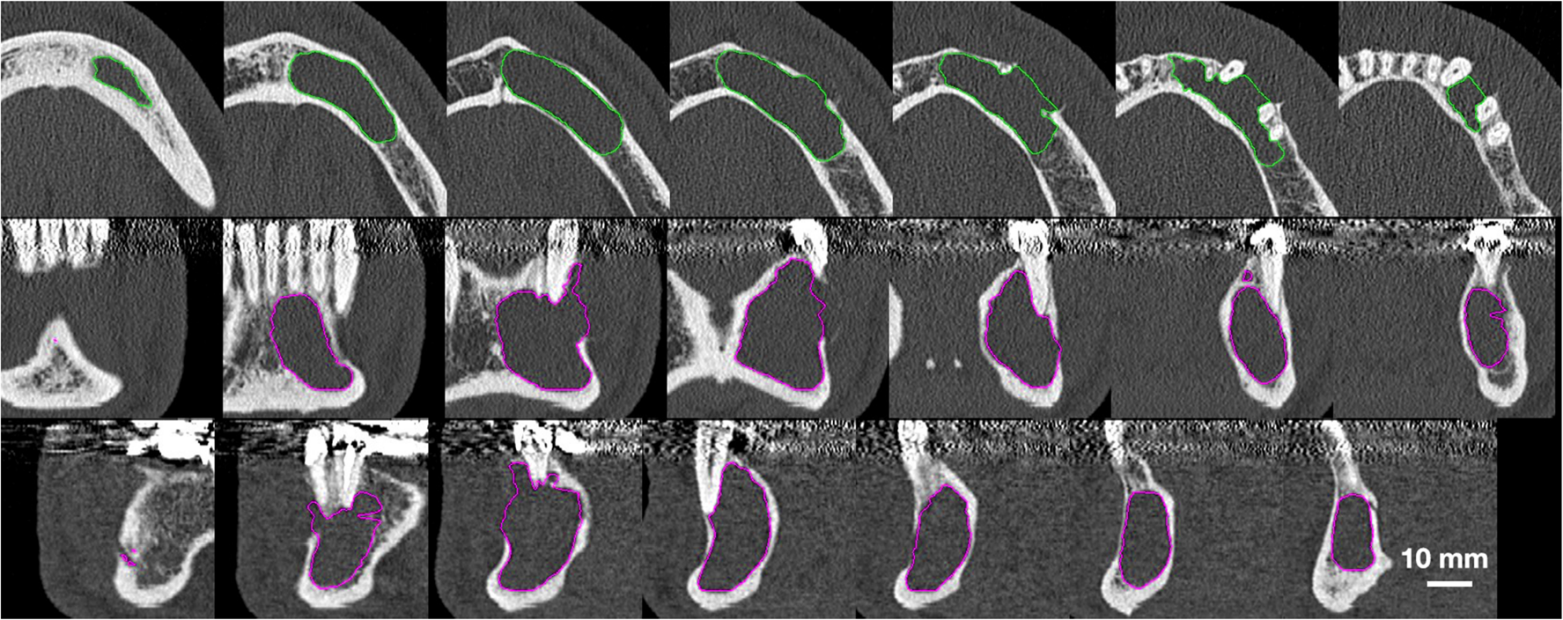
Radiographic analysis. Representative samples from the radiographic data markup process. First row manual localization of the cyst (neon green) on the axial slices, second and third rows display of automated reconstruction (magenta) based on prior manual localization
